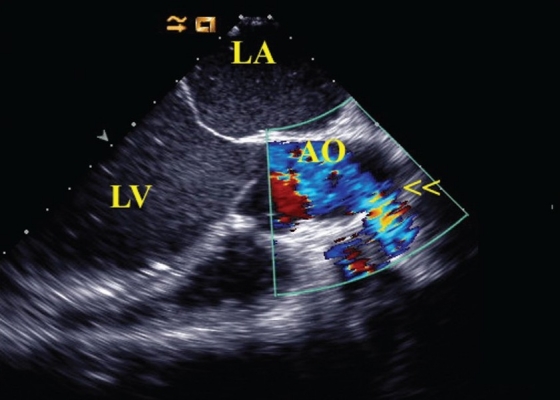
Intraoperative transesophageal echocardiography at 115°, showing the outflow conduit of the LVAD into the ascending aorta (arrows)
